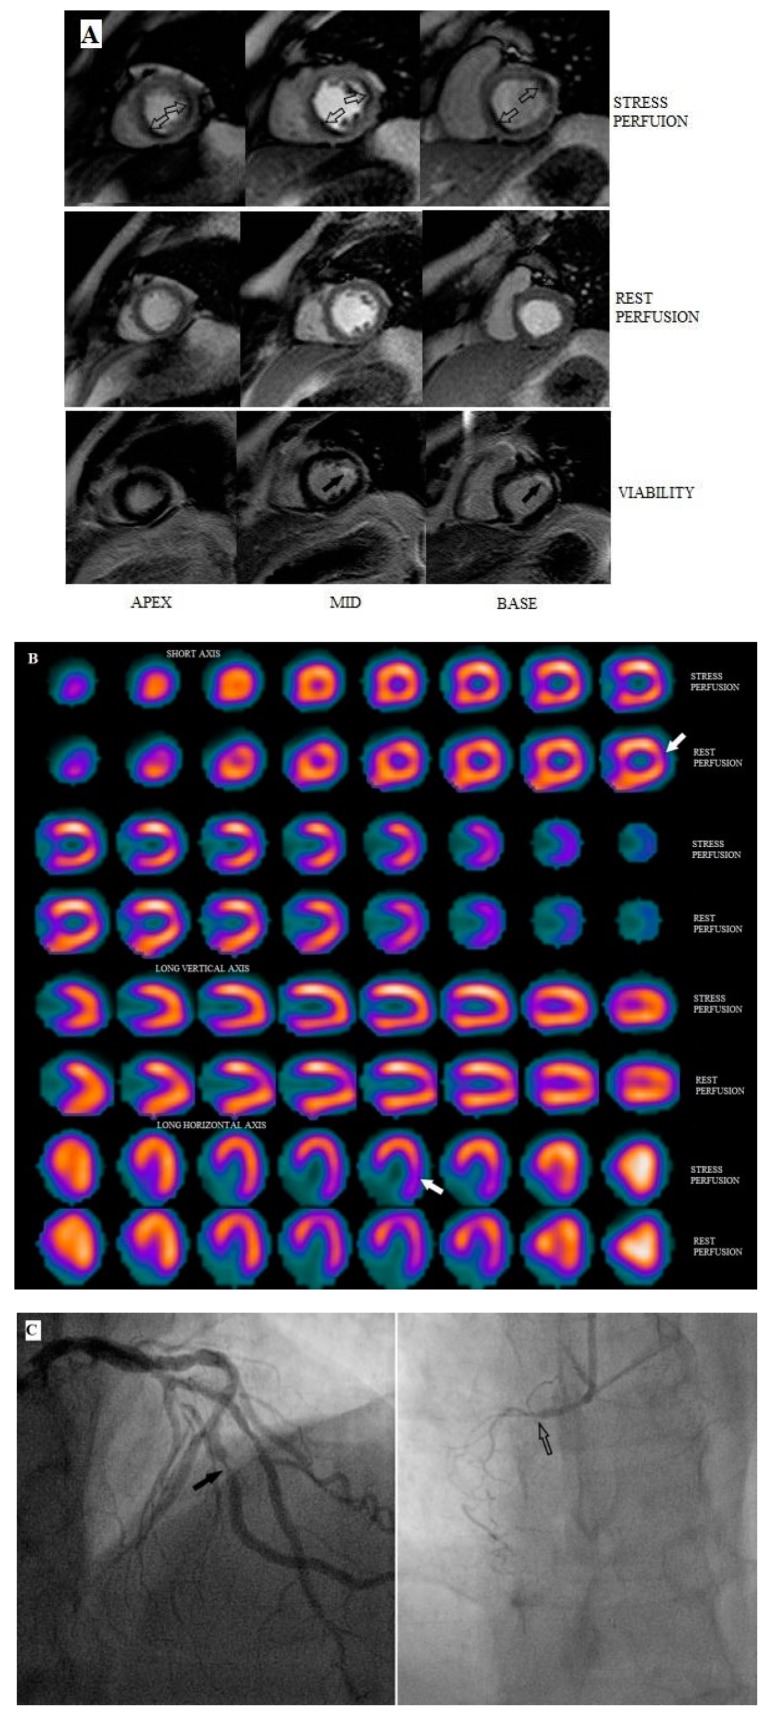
Representative images from a 72 year-old male patient with chest pain. (A) Stress-rest cardiac magnetic resonance (CMR) images show stress-induced perfusion defects (ischemia) in the lateral-anterolateral and inferoseptal left ventricular walls of the basal, mid and apical slices (open arrows) and subendocardial late gandolinium enhancement (LGE) (non-transmural infarction) in the anterolateral wall at the basal and mid-ventricular slices (black arrows). (B) single-photon emission computed tomography (SPECT) shows a partially reversible defect (white arrows) at the lateral and anterolateral left ventricular walls, but fails to demonstrate the inferoseptal ischemia. (C) SCA demonstrates a significant (>70%) luminal stenosis (left panel, black arrow) at the obtuse marginal branch and occlusion of the right coronary artery (right panel, open arrow) confirming the CMR findings.
